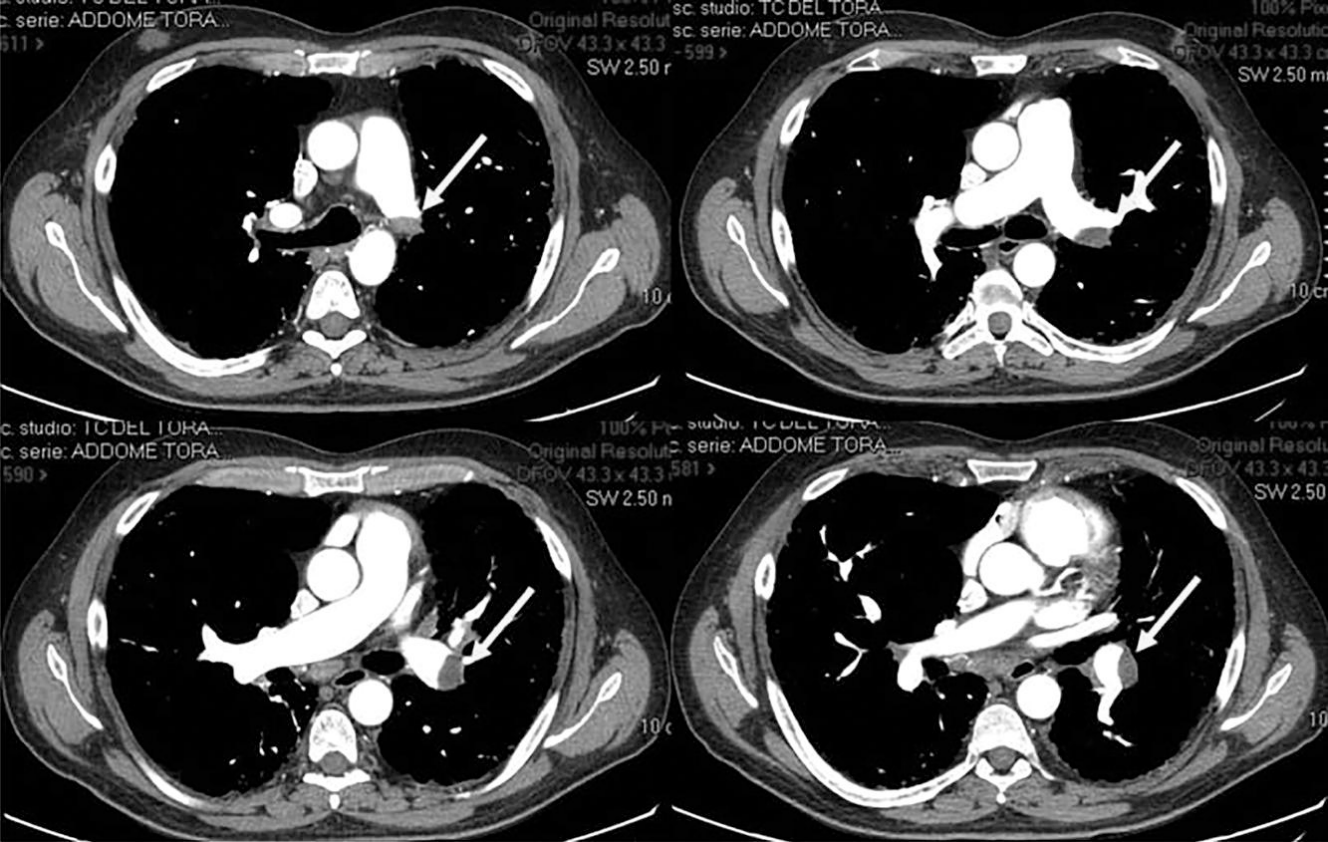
Computed tomography scan of the chest. After administration of contrast medium, partially occluding thrombosis was shown in left pulmonary artery and its main branches.
